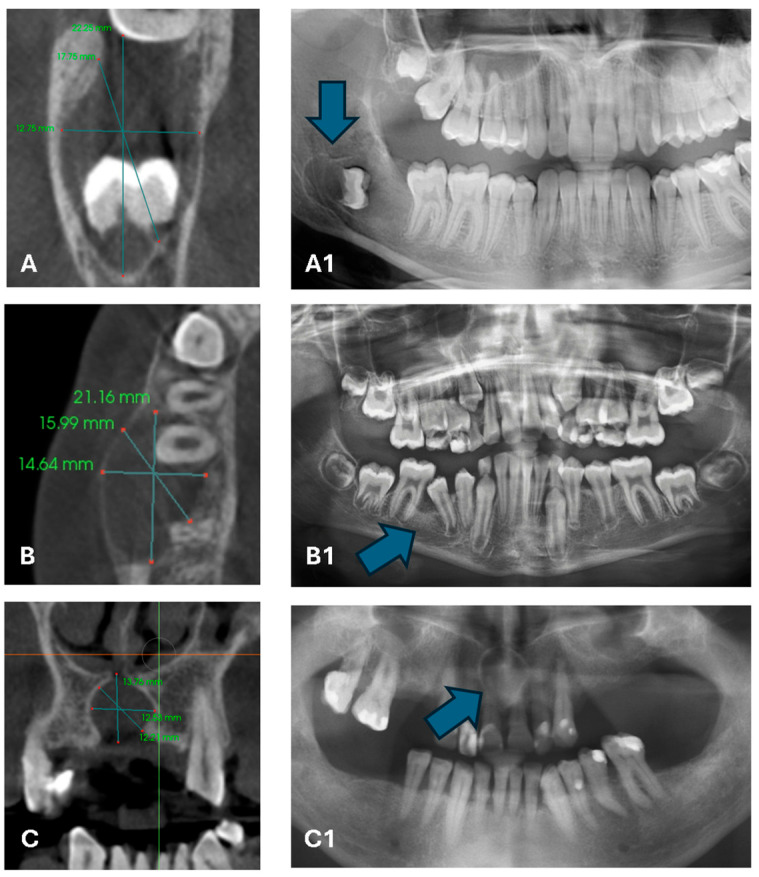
Representative imaging features of odontogenic cysts on cone-beam computed tomography (CBCT) and panoramic radiography. (A,A1) Dentigerous cyst: (A) CBCT showing a well-defined radiolucent lesion associated with the crown of an impacted tooth; (A1) corresponding panoramic radiograph. (B,B1) Odontogenic keratocyst: (B) CBCT demonstrating a unilocular radiolucency with characteristic dimensions; (B1) corresponding panoramic radiograph. (C,C1) Radicular cyst: (C) CBCT revealing a periapical radiolucent lesion; (C1) corresponding panoramic radiograph.
